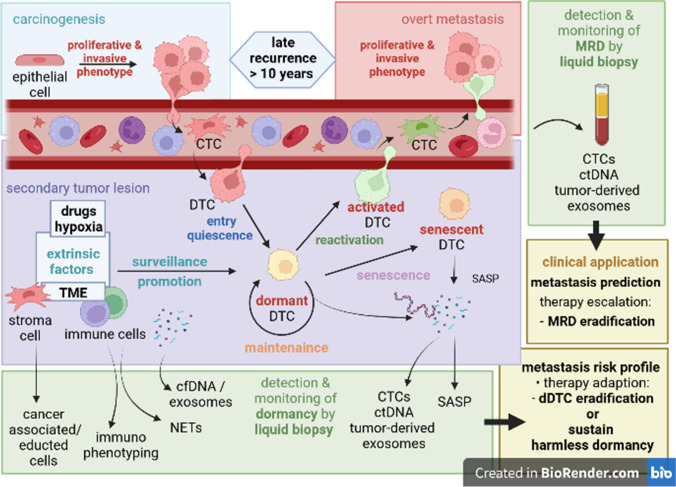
Dormancy. Dissemination and tumor dormancy—MRD and dormancy biomarkers indicating late tumor recurrence. TME tumor microenvironment, CTC circulating tumor cell, ctDNA circulating tumor DNA, MRD minimal residual disease, NETs neutrophil extracellular traps, SASP senescence-associated secretory phenotype, DTC disseminated tumor cell, dDTC dormant disseminated tumor cell. During carcinogenesis, tumor cells undergo different adaptions enabling malignancies to invasive growth, escape of immune surveillance, epithelial–mesenchymal transition, and induction of tumor vascularization. Often, intravasation and dissemination were achieved before solid malignancies were clinically captured or have been detected by radiographic diagnostics. Minimal residual disease (MRD) tracking with liquid biopsy might be a more suitable diagnostic instrument for detection of early blood dissemination. Enhanced by metastatic niche preparation, disseminated tumor cells (DTC) extravasate and colonize secondary organs and form immediately overt metastasis. However, some entities tent to endure in a tumor dormant status. Previously invasive and proliferative tumor cells switch therefore into a quiescent cell state in which these cells resist immunity for years. Driven by a plethora of partly unknown processes and programs, dormant disseminated tumor cells (dDTCs) awake and re-gain proliferative phenotypes. For early prediction of disease progression, there is an urgent need for the exploration and clinical introduction of blood-based liquid biopsy biomarkers indicating early dynamics in MRD. On the other side, detection of dormant DTCs resting in cell cycle arrest and in metabolic quiescence circumventing invasive procedures like bone marrow punctation might be challenging and requires complementary and comprehensive tumor-derived and microenvironment biomarkers
